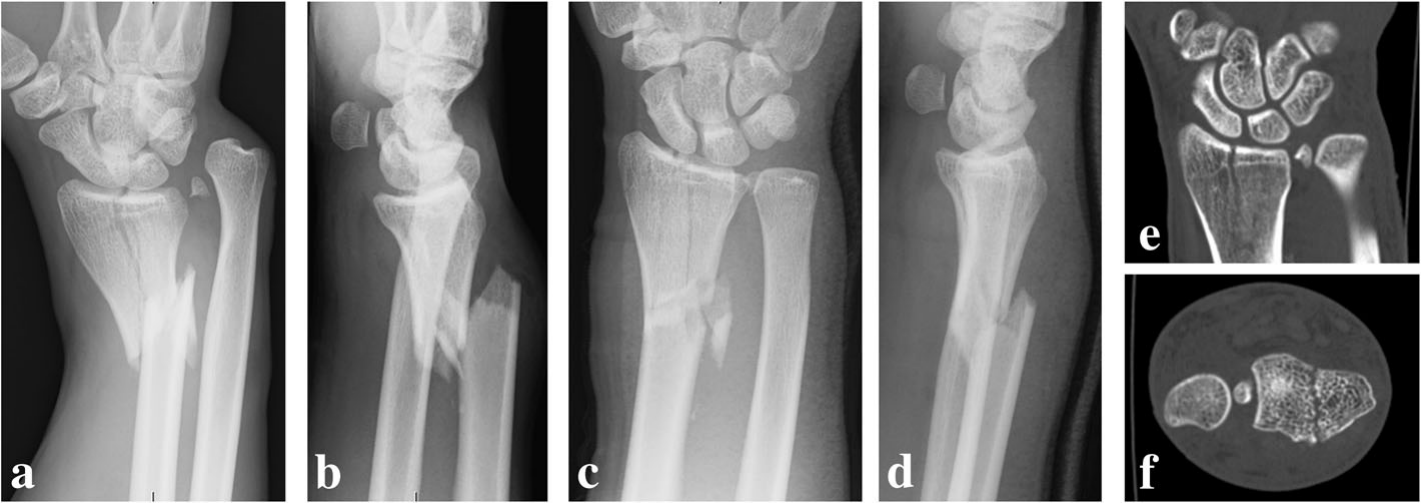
Plain radiograph and plain computed tomography (CT) scan of the right wrist before and after manual reduction. (a) Anteroposterior (AP) view and (b) lateral view before manual reduction. (c) AP and (d) lateral views after manual reduction. (e) Coronal view and (f) axial view of the CT image of the distal radioulnar joint. The fragment of the ulnar styloid is trapped between the sigmoid notch and ulnar head
